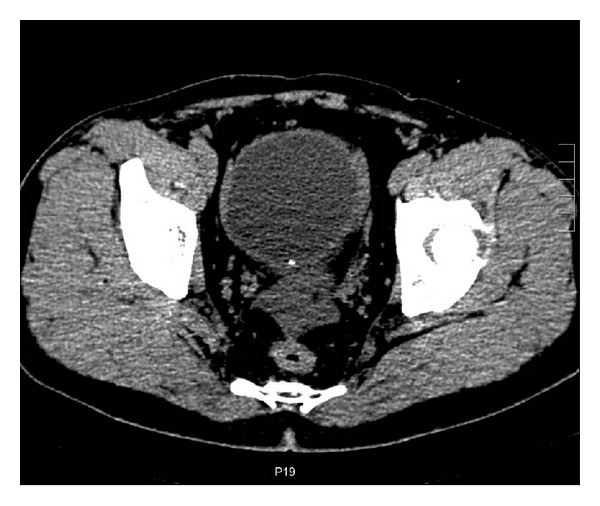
Axial CT shows cystic wall with punctate calcification in the distal portion.
